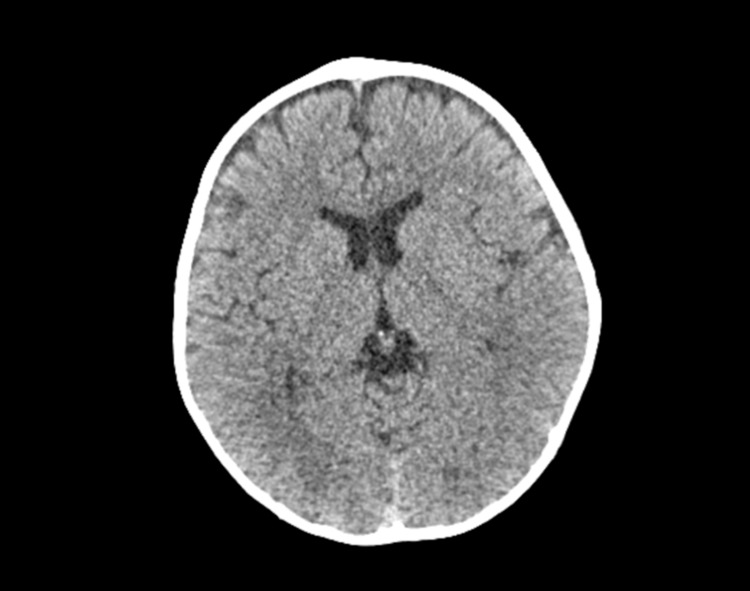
CT findings consistent with PRES (patient 2) Bilateral occipital hypodense white matter lesions on the parieto-occipital regions.
